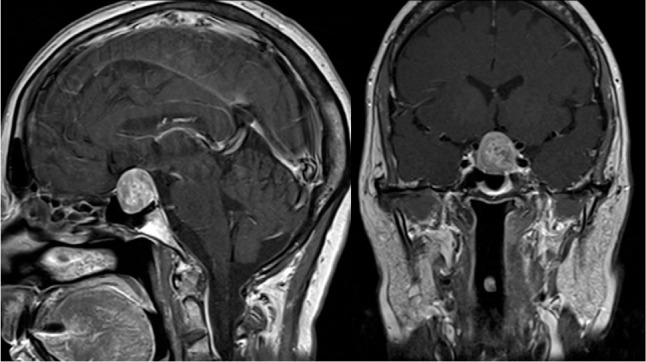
Sagittal and coronal views of T1 MRI with gadolinium showing a
